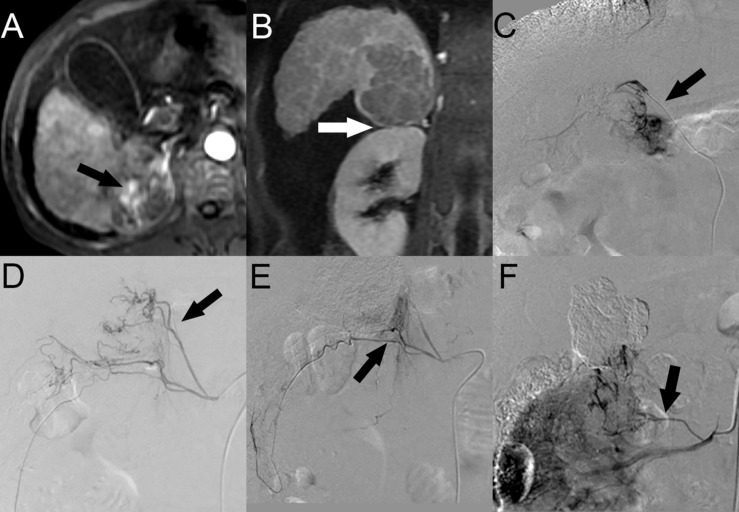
HCC with SARPS invasion in a 49-year-old man. (A) Contrast-enhanced arterial phase MR image showed a 5.4-cm-sized enhanced tumor (black arrow) in segment 7 of the right lobe of the liver in the axial plane. (B) Portal phase MR image showed clear tumor invasion of the SARPS in the coronal plane (white arrow). (C) Angiographic image at the first TACE session revealed that the tumor was supplied by the posterior branch of the RIPA (black arrow). (D) Angiographic image at the second recurrence revealed that the EHCs were supplied by the inferior branch of the RAA (black arrow). (E) After the inferior branch of the RAA was successfully chemoembolized, the angiogram showed that the superior branch of the RRCA was also involved in the tumor blood supply (black arrow). (F) Angiographic image at the fourth recurrence revealed that the tumor was supplied by the new inferior branch of the RAA (black arrow). Abbreviations: HCC, hepatocellular carcinoma; SARPS, superior aspect of the right perirenal space; RIPA, right inferior phrenic artery; RRCA, right renal capsular artery; RAA, right adrenal artery; and EHC, extrahepatic collateral vessel.
